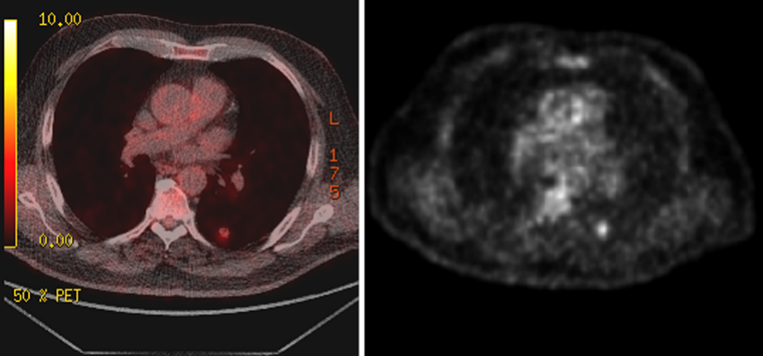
Fused PET/CT image (*left*) and non-attenuated corrected PET image (*right*) demonstrating an intense FDG uptake in a coccidiodoma seen in the left lung
